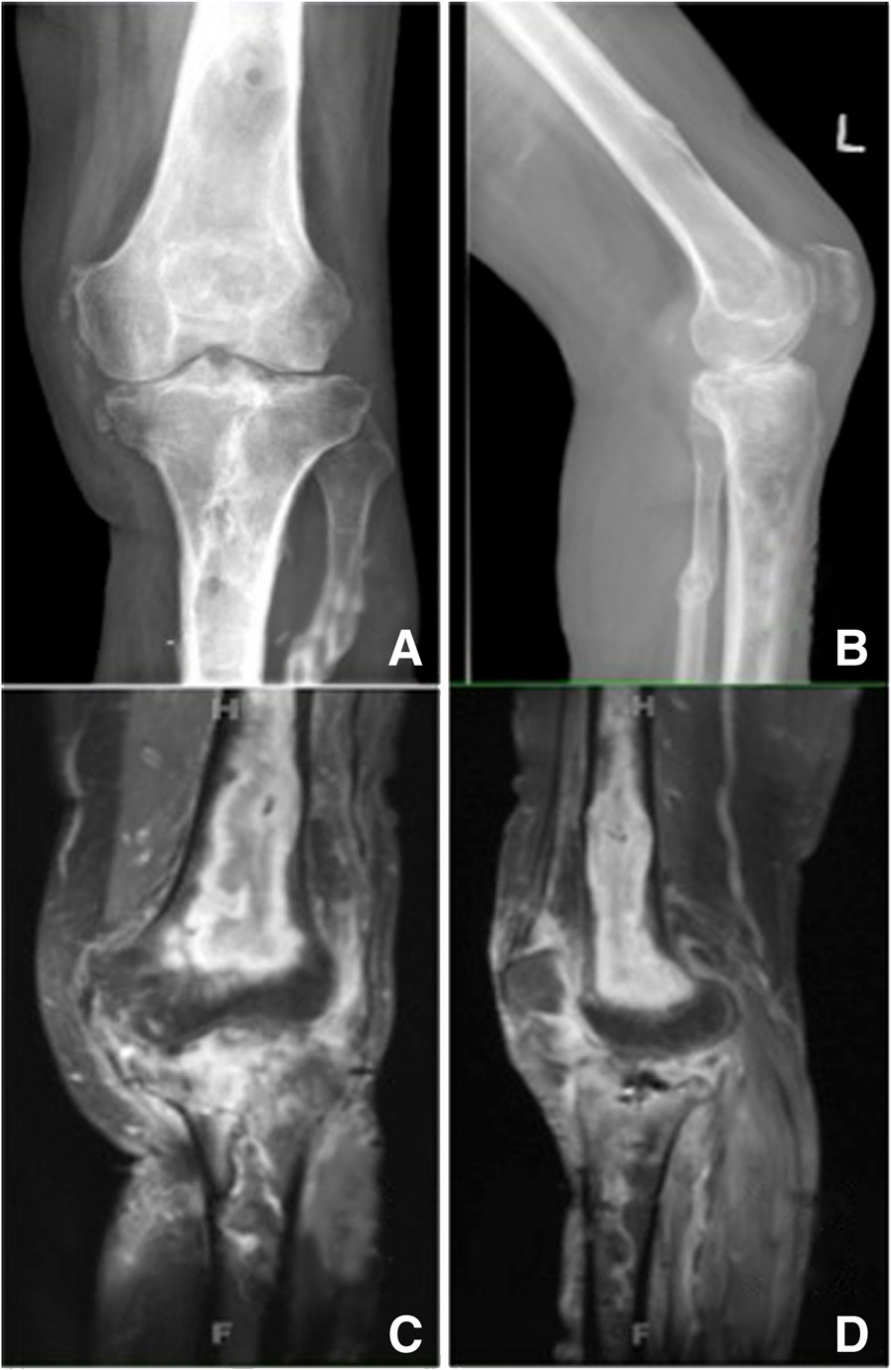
Preoperative radiographs and MRI scans of the affected femur. Pre-Operative Images: Anterio-posterior (A) and lateral (B) X-ray of a left leg revealing a large lytic lesion of the distal femur. In (C) and (D) coronal and sagittal STIR MRI sequences revealing the distal femoral osteolmyelitis.
